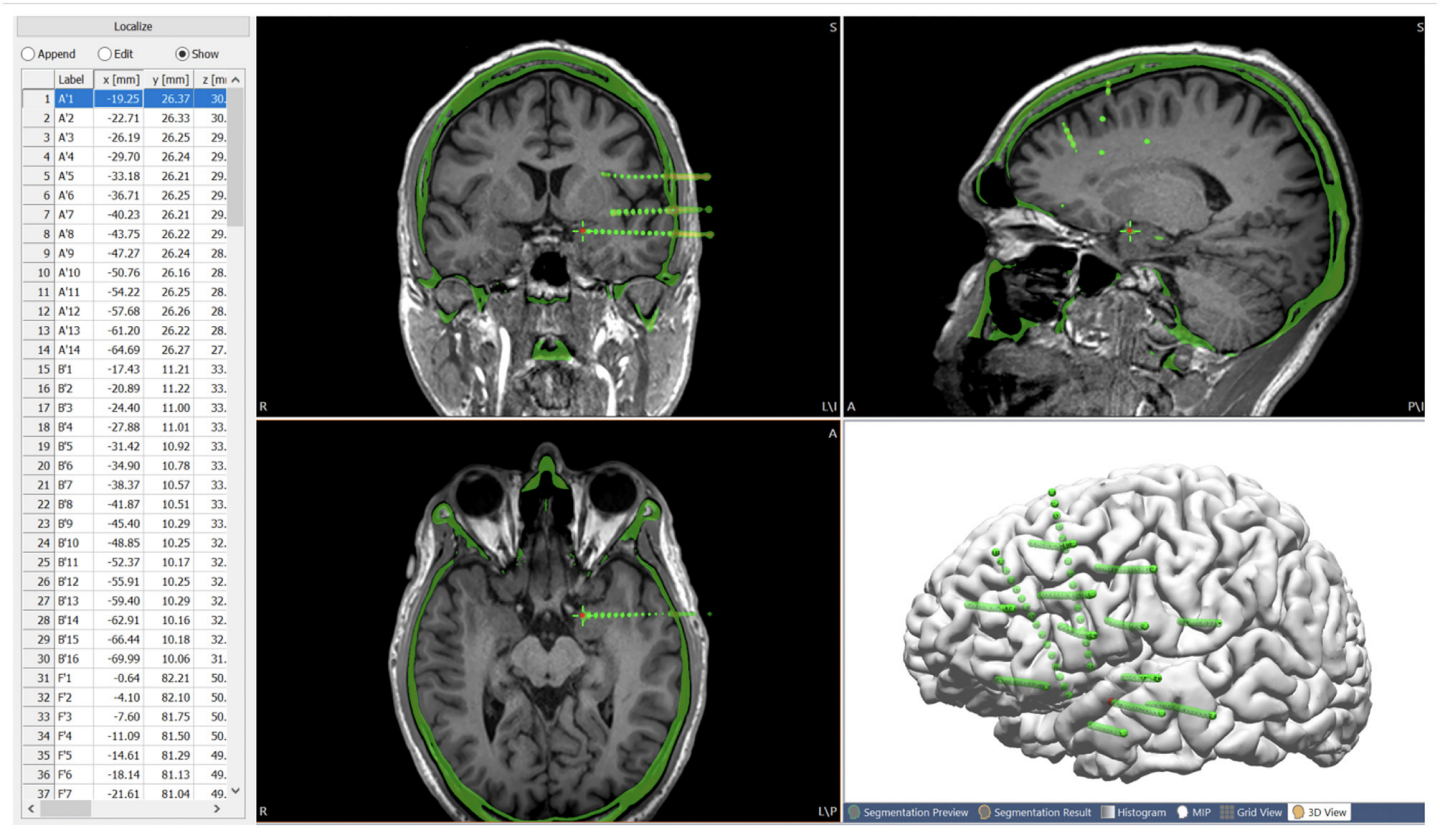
Post-SEEG-implantation CT images co-registered with the T1-weighted base image, with all the electrode contacts segmented from the CT (center of highest intensity) and stored as a list of localize points with coordinates for the electrode contacts. Green spheres indicate extracted electrode contacts. Red contact indicates the current electrode contact (highlighted on the list of localize points), the location of which is synchronized on the MRI and CT.
